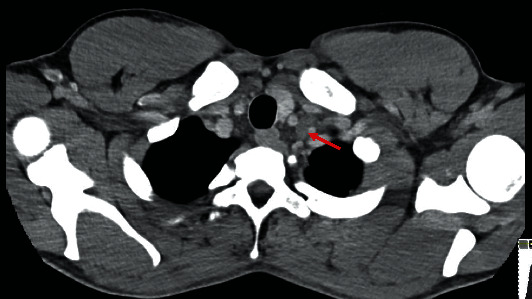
CT. Axial thoracic contrast-enhanced CT image at the thoracic inlet, showing a left supraclavicular lymphadenomegaly (red arrow).
